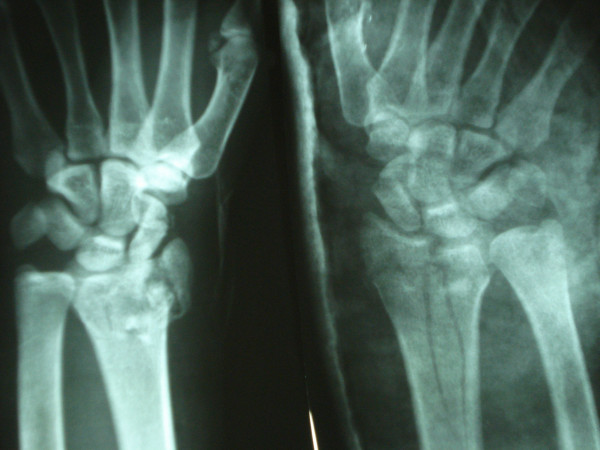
Preoperative AP roentgenogram of the right and left distal radius and scaphoid fractures.
